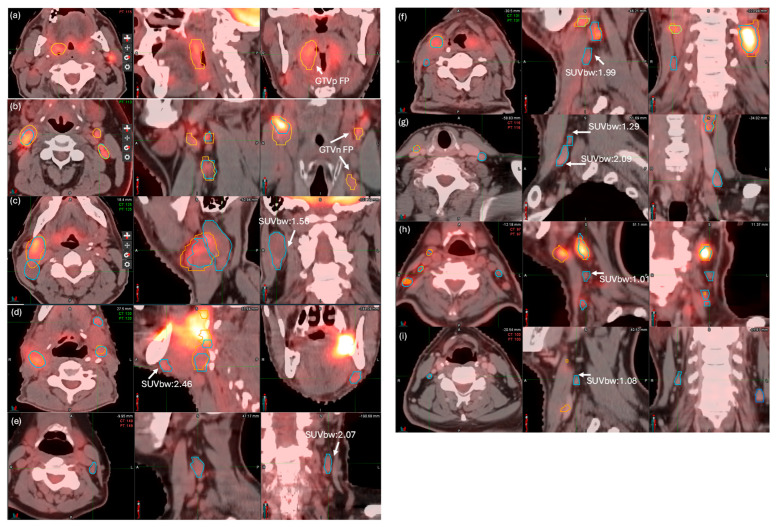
Example cases of false-positive errors for GTVp (a) and GTVn (b) and false negative errors for GTVn with small SUV value (c–i) (golden contour indicates DLAS nodes, light blue indicates RadOnc manual contour).
